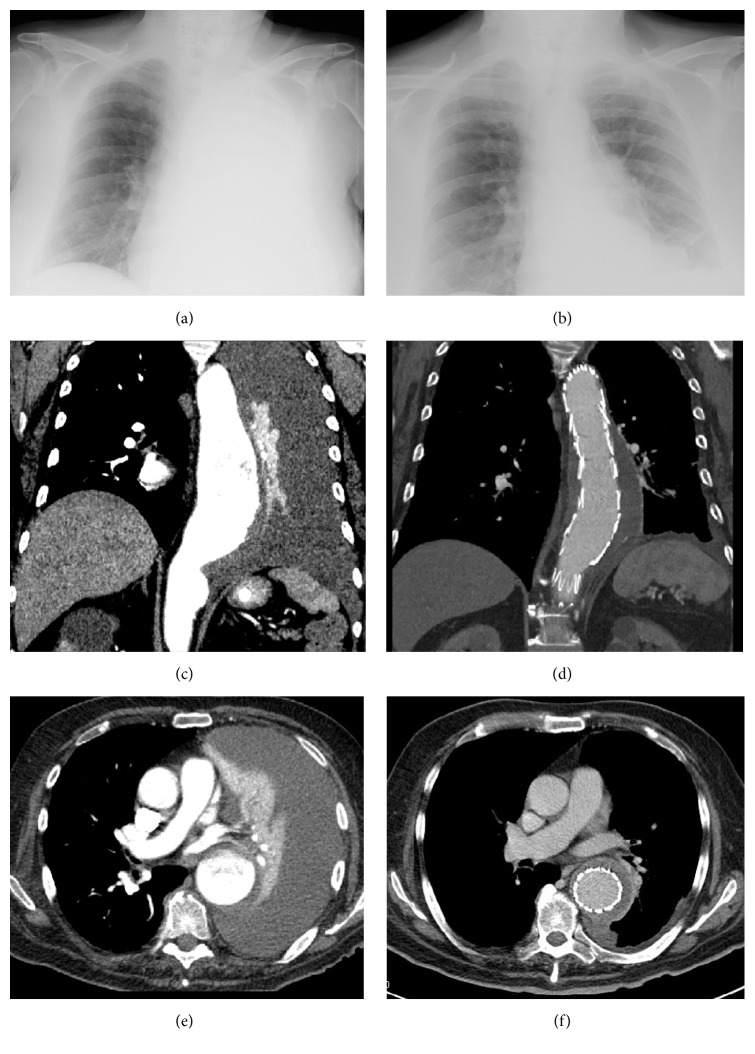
Chest X-ray before (a) and after (b) endovascular repair of thoracic aortic aneurysm (TEVAR) showing the complete opacity of the left lung field due to aneurysm rupture within the pleura (a) and the complete resolution after blood drainage (b). CT-scan before (c and e) and after (d and f) endovascular repair of thoracic aortic aneurysm by TEVAR.
